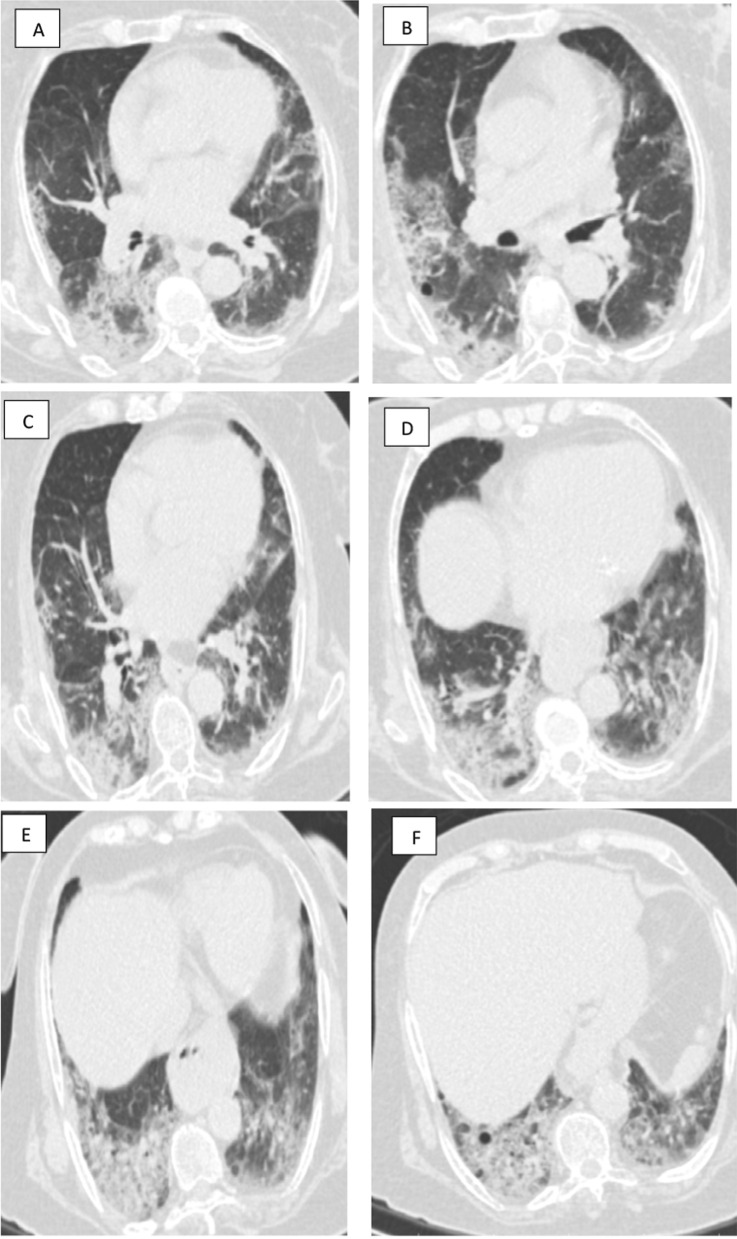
A 52-year-old infected doctor with COVID-19 presented with dyspnea and on low flow oxygen support. A, B Axial chest CT cuts (lung window) showing multiple bilateral sub-pleural patchy areas of ground glass attenuation and sub-segmental consolidations in both upper lobes (20%). C, D Axial chest CT cuts (lung window) showing involvement of middle lobe and lingula (5–10%). E, F Axial chest CT cuts (lung window) showing involvement of right and left lower lobes (80% and 75%%) by patchy sub-segmental consolidative areas, curvilinear band and ground glass pattern, interstitial thickening, reticulo-nodular infiltrates and fibrosis, mild bronchial and vascular dilatation. CT severity score (CTSS) is 16/25
